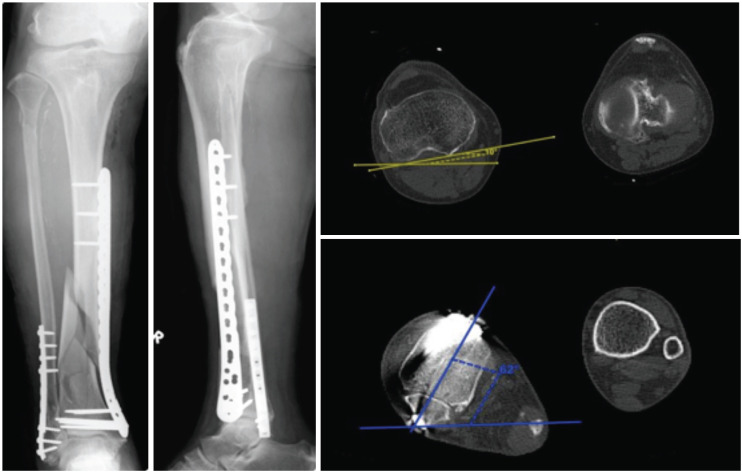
Post-operative radiographs of A3 distal tibia fracture with 45° of malrotation. Post-operative radiographs and Computed tomography scans which demonstrated 52° of external rotation of the distal joint block relative to the posterior tibia condyles. The uninjured limb was in 7° of external rotation with a resultant malrotation of 45° of external rotation.
